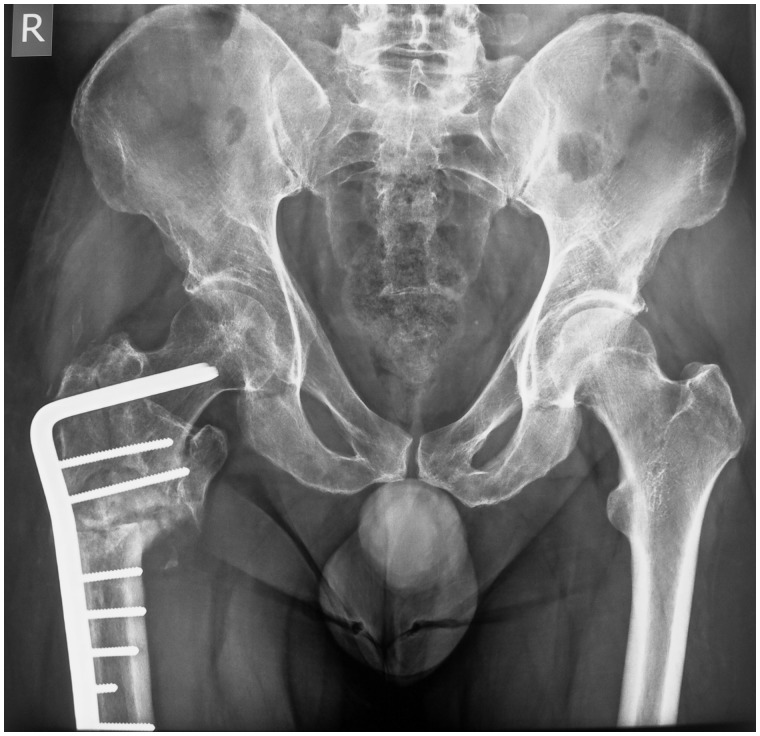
Anteroposterior pelvic radiograph following a valgus-derotational subtrochanteric osteotomy of the right hip for a malunited peritrochanteric fracture with involvement of the lesser trochanter (LT). The original injury and the valgus component of the corrective osteotomy have brought the right LT in close proximity to the ischium. Such a patient would be at risk for ischiofemoral impingement.
